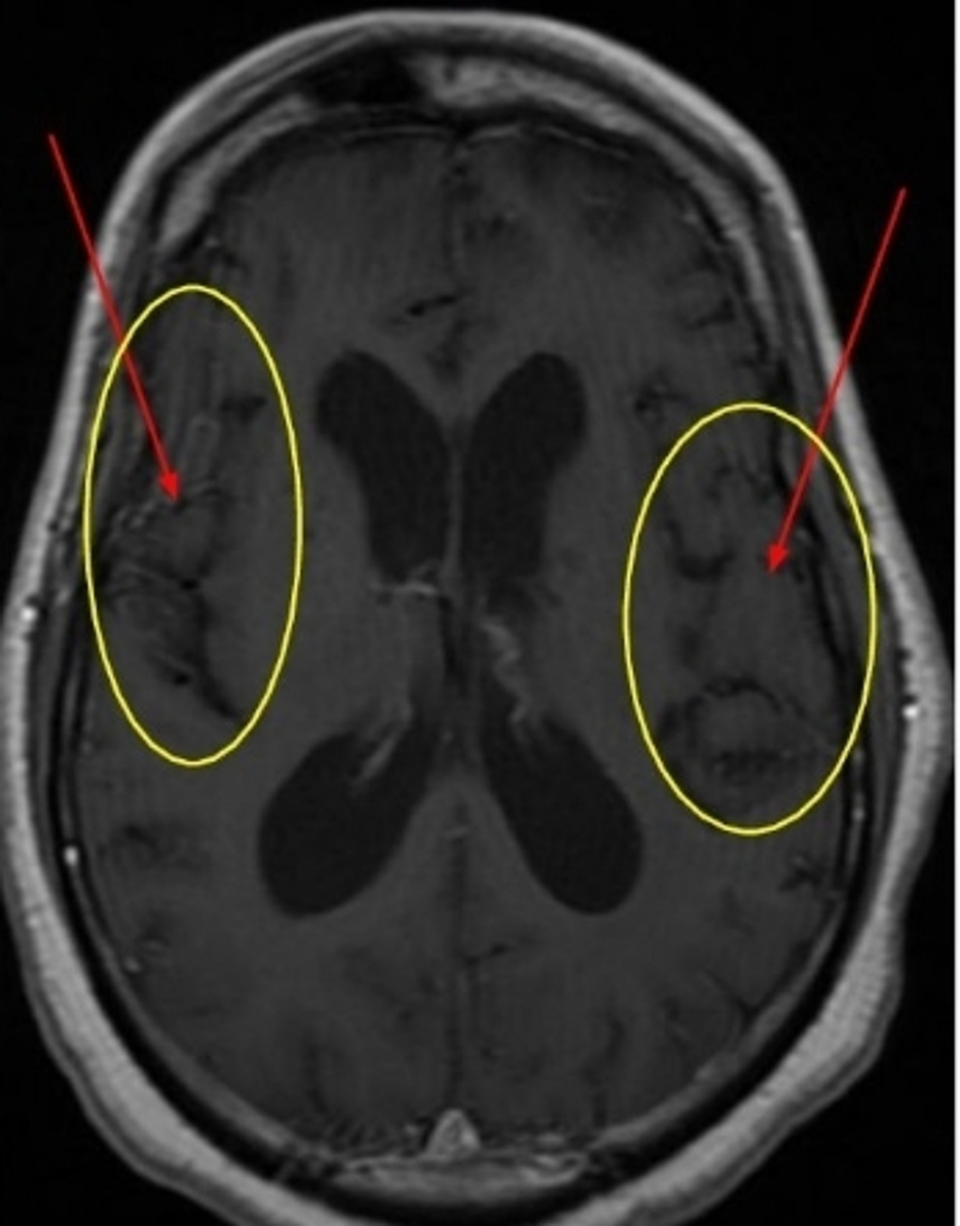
Brain MRI, T1 with gadolinium Bilateral temporal lobe atrophy. Circle: temporal lobe, Arrows: temporal lobe atrophy.
